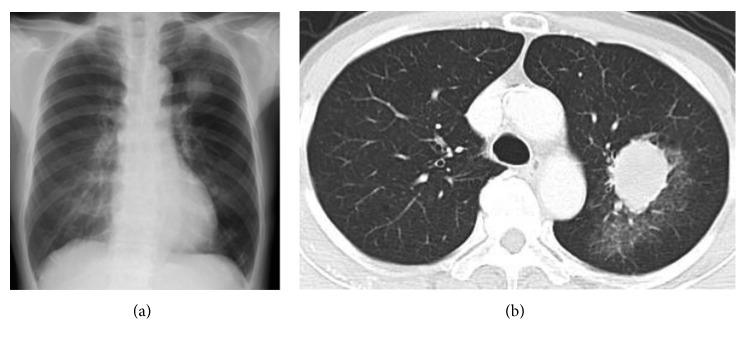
Chest X-ray showing an apparent nodule in the left upper lung field (a). Contrast-enhanced computed tomography of the chest, showing a large nodule with surrounding ground-glass opacity in the left upper lobe of the lung (b).
